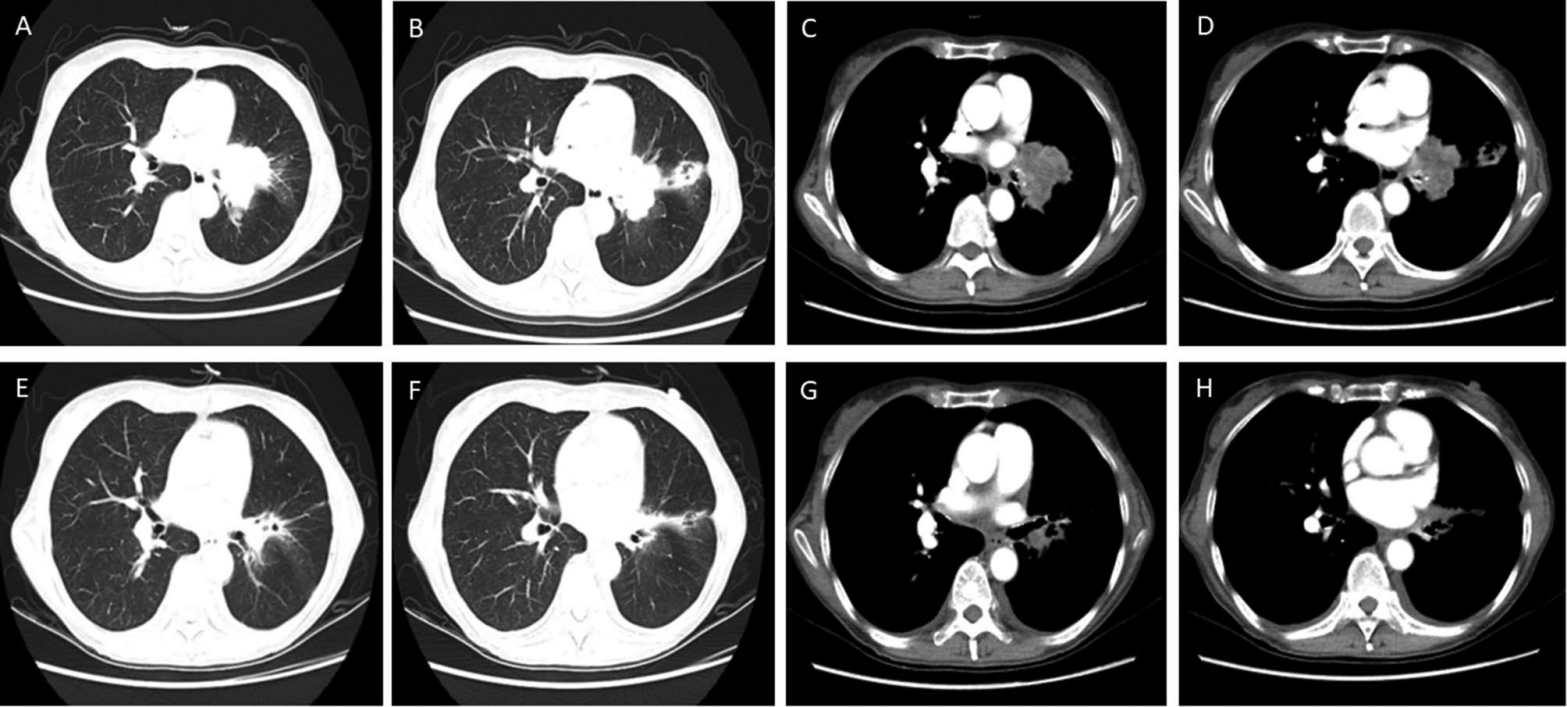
Contrast-enhanced chest CT scan before and after crizotinib treatment. Panels A-D: Prior to crizotinib treatment. Panels E-H: Post 2 months on crizotinib treatment, showing the size of primary tumor on left hilum significantly decreased
